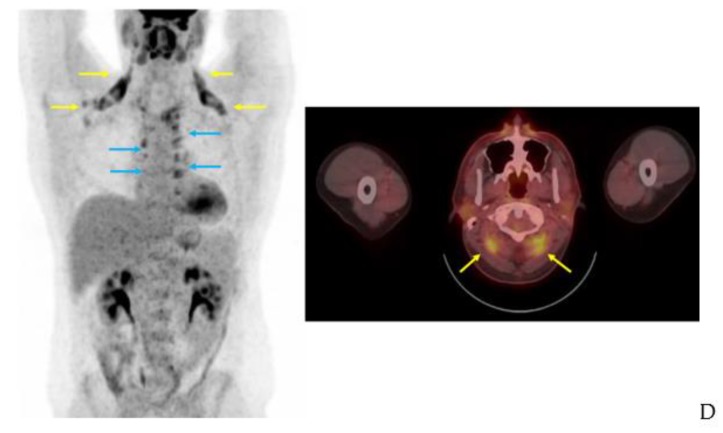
Variation of BAT FDG uptake in clinical patients. Fused coronal FDG-PET/CT, coronal CT, and fused axial FDG-PET/CT (A–C). (A) BAT FDG uptake in a woman with normal BMI. Note supraclavicular (yellow arrows), skull base (red arrow), paraesophageal (blue arrow), and perinephric (white arrow) uptake. (B) Supraclavicular BAT in an obese woman (yellow arrows). (C) Diffuse FDG uptake in subcutaneous WAT in a male patient (yellow arrows). (D) A male patient with extensive supraclavicular FDG uptake extending into the upper neck (yellow arrows) and paravertebral BAT activity (blue arrows).
